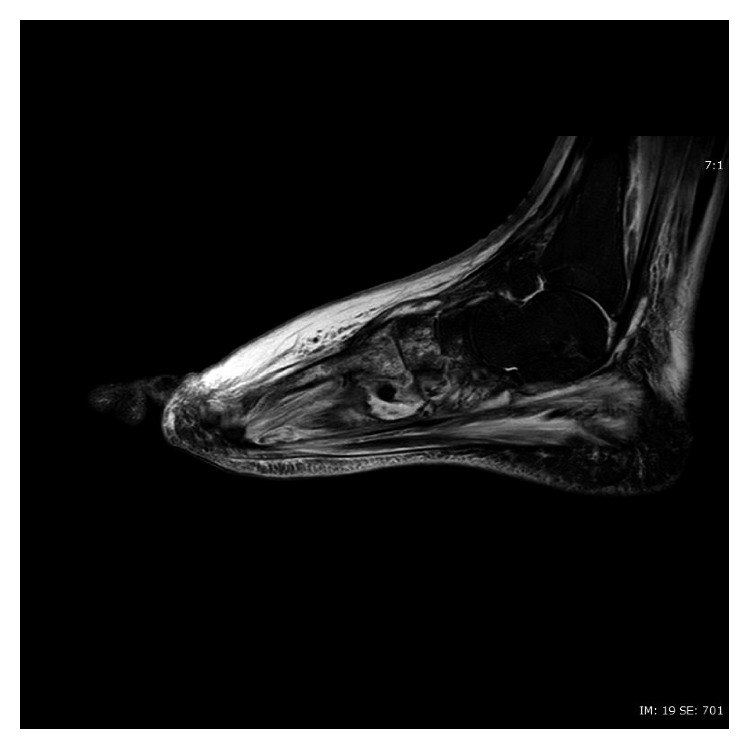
T2-weighted sagittal image of MRI of left foot without contrast, showing cellulitis of soft tissues, as well as deep-seated multilocular collection at Lisfranc's joints and second metatarsophalangeal joints.
